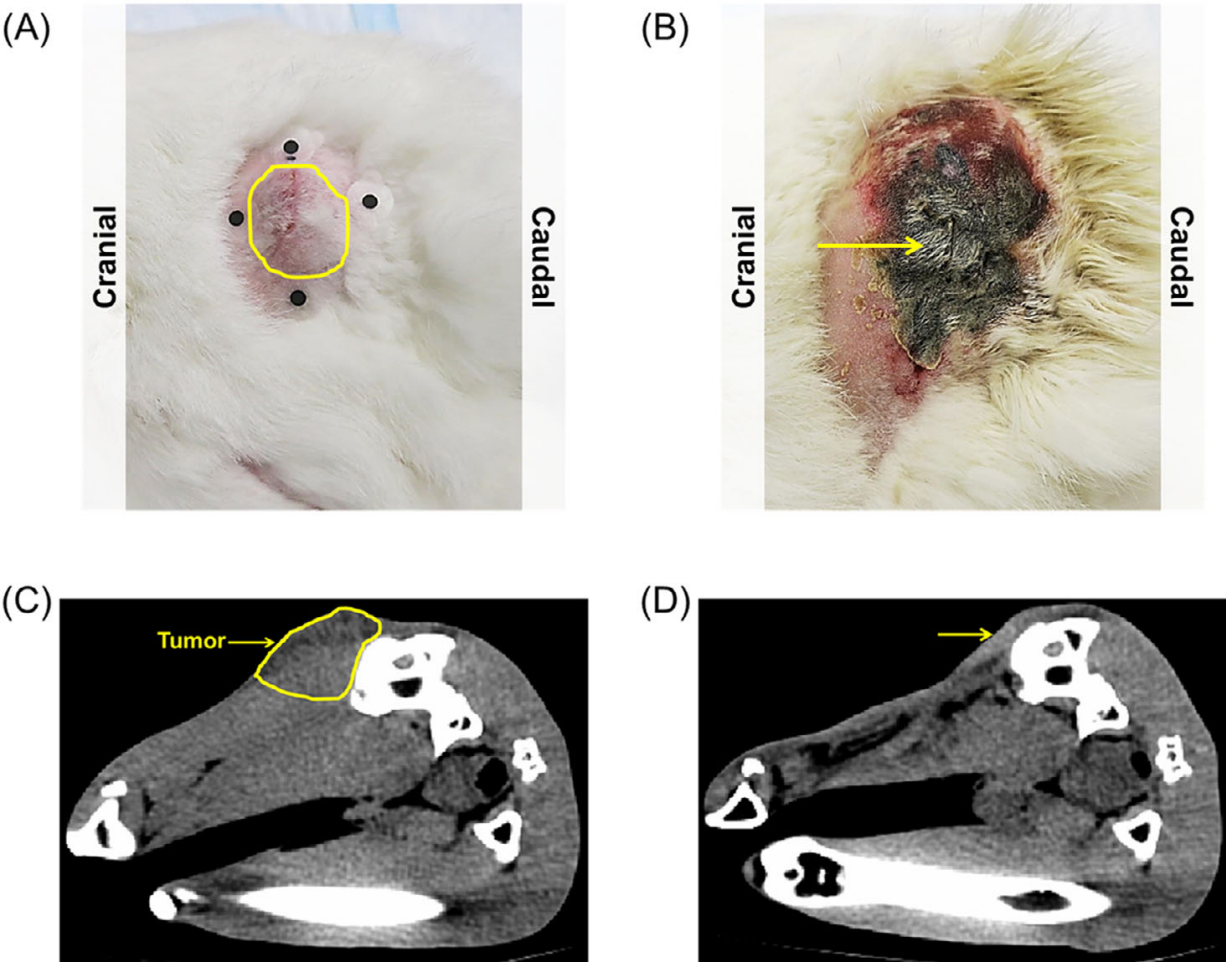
RB2018‐30 tumor response after Photofrin^®^‐mediated I‐PDT. (A) Image of the tumor 1 day prior to treatment. (B) Image of tumor 6 days post‐treatment. (C) and (D) are, respectively, CT scans of the tumor 1 day prior to and 13 weeks post‐treatment. The yellow arrow indicates where the tumor was located in the CT scans.
